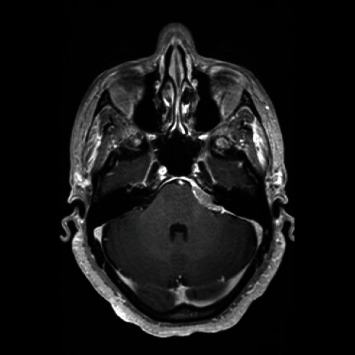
Post contrast MRI demonstrates a well-defined homogeneously enhancing extra-axial dural-based mass in the left CP angle suspicious for an en plaque meningioma.
